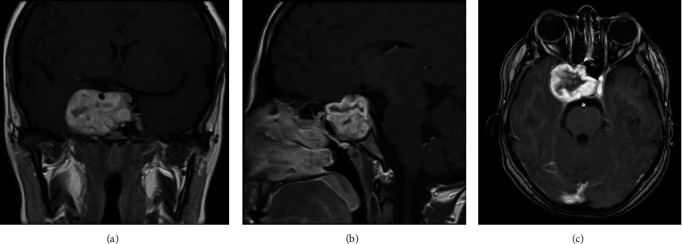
(A–C) Coronal, sagittal, and axial brain MRI with contrast showing a well-defined heterogeneously enhancing lesion in the sellar region measuring about 4.5 cm × 4 cm × 4 cm. It is displacing the medial surface of the right temporal lobe and compressing on the right optic nerve and optic chiasm. The right internal carotid artery is completely encased by the lesion and displaced superiorly. MRI, magnetic resonance imaging.
